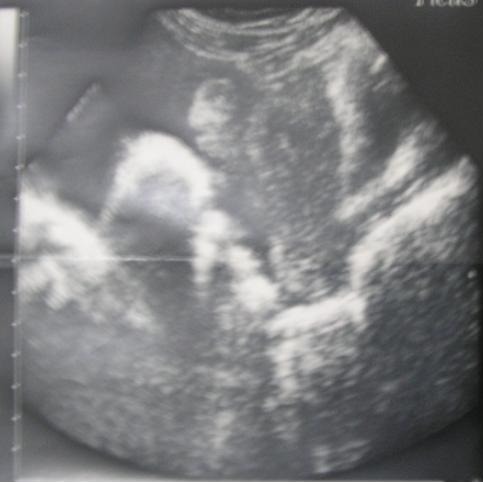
Abdominal pelvic ultrasound in longitudinal section Heterogeneous mass behind uterus with 4 centimeters of biggest diameter
